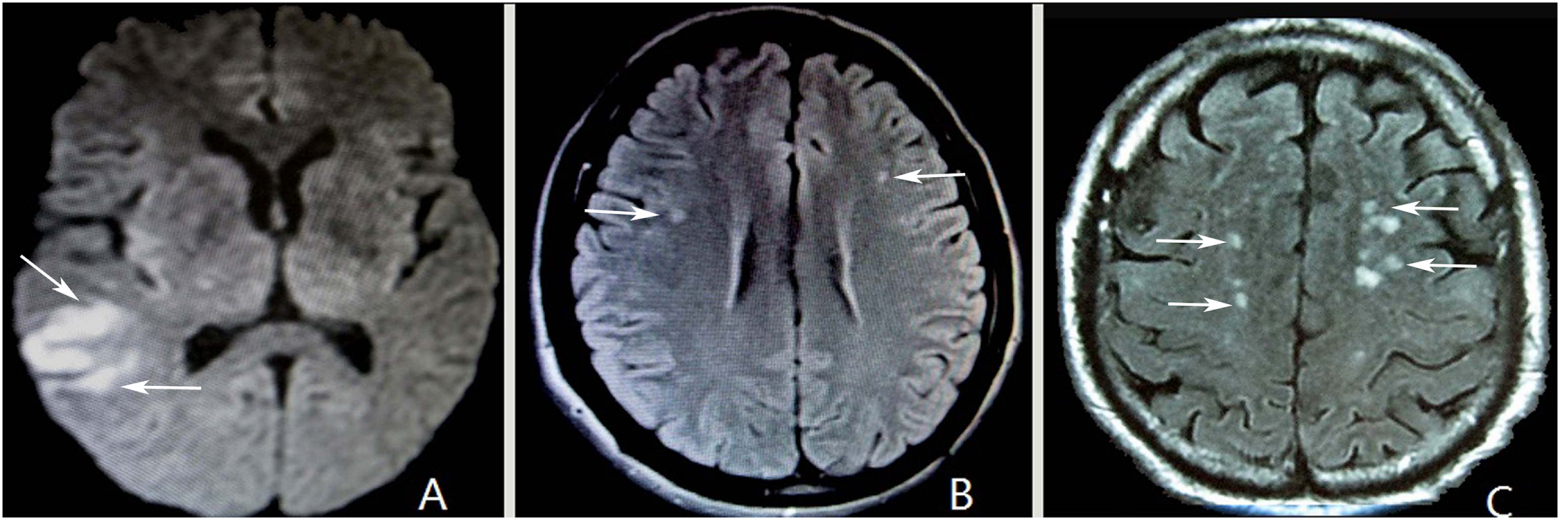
Nonfocal symptoms in MIS patients with or without MetS on brain MRI. A 65-year-old female with headache and dizziness for 3d (NIHSS = 0 score), several typical small infarcts in the right lateral temporal and parietal area is visible on the DWI (A, arrows), and she were diagnosed with MIS with MetS. A 52-year-old male with dizziness for 2 d (NIHSS = 0) and with MetS, on the FLAIR imaging shows two recent small infarcts near the centrum ovale areas (B, arrows). A 76-year-old male with recurrent headache for 10 d (NIHSS = 0) without MetS, MRI-FLAIR shows typical recent multiple small infarcts in bilateral anterior circulation areas (C, arrows).
